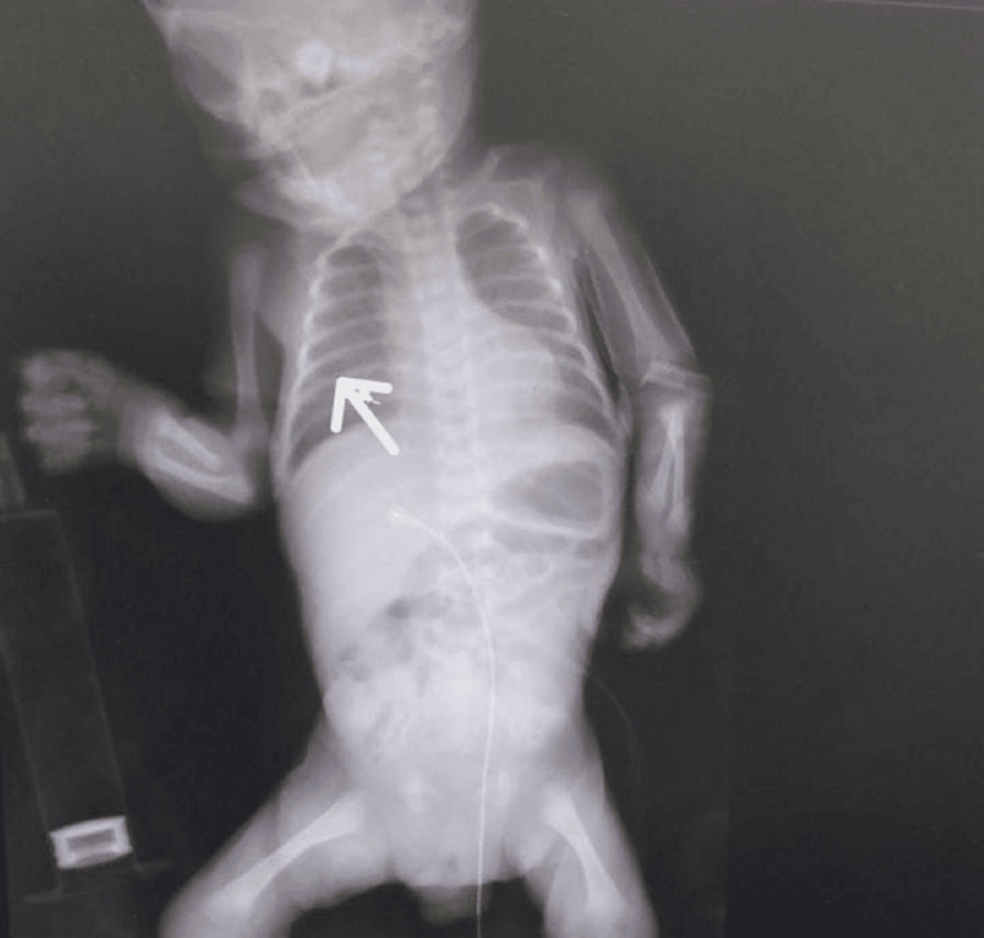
X-ray showing normal lung fields after the removal of the umbilical venous catheter
